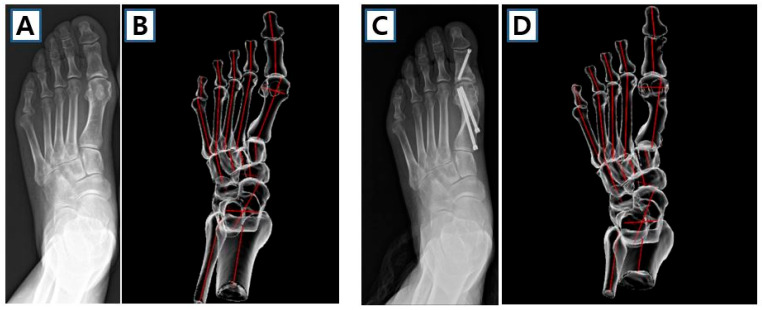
WBCT-based automated 3D analysis of hallux valgus deformity (A,B) before and (C,D) after surgery. (A) Preoperative weightbearing anteroposterior radiograph demonstrating a moderate hallux valgus deformity. (B) Preoperative automated 3D analysis showing the bone axes used for alignment assessment. (C) Weightbearing anteroposterior radiograph obtained 12 months after minimally invasive hallux valgus correction. (D) Postoperative automated 3D analysis demonstrating the reconstructed bone axes (red lines) and corrected alignment.
